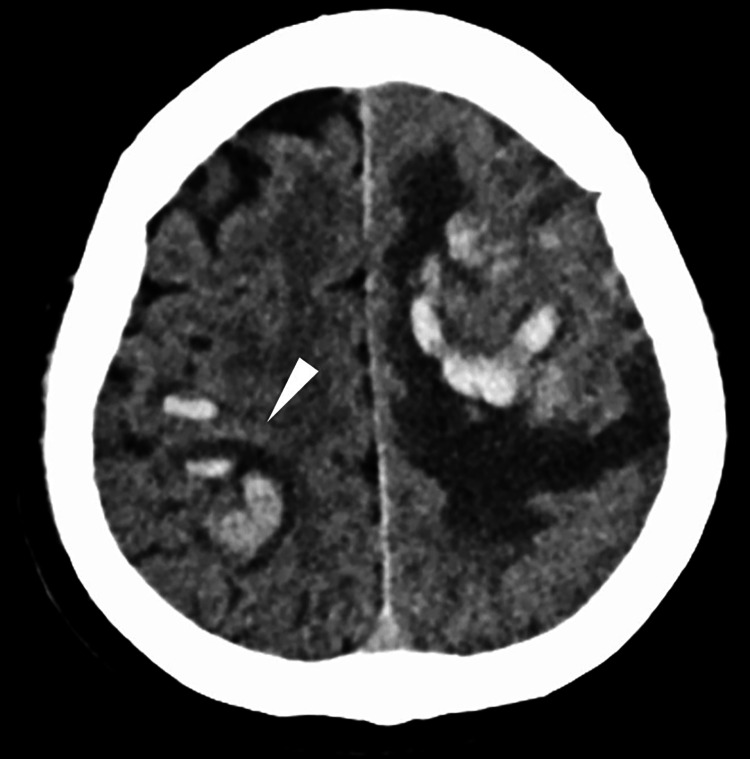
CT seven days after admission Image showing a new intracerebral hemorrhage in the right parietal lobe (white arrowhead).
